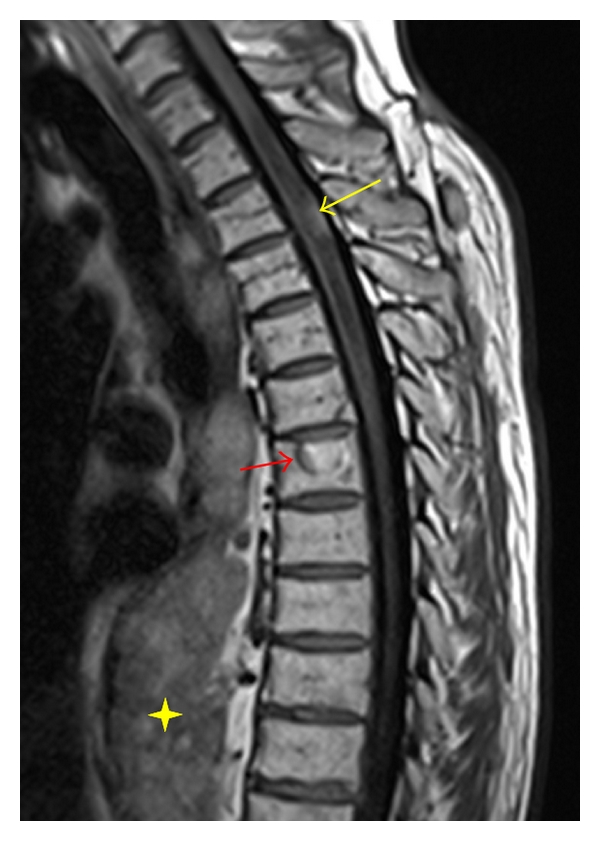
Sagittal T1-weighted MR image reveals an intramedullary hyperintense lesion in the dorsal upper thoracic cord from a melanoma metastasis (yellow arrow). There is extensive surrounding hypointensity due to spinal cord edema. Additionally, there is a T1 hyperintense vertebral body metastatic lesion (red arrow). Large lobulated paraspinal masses are also noted (yellow star).
